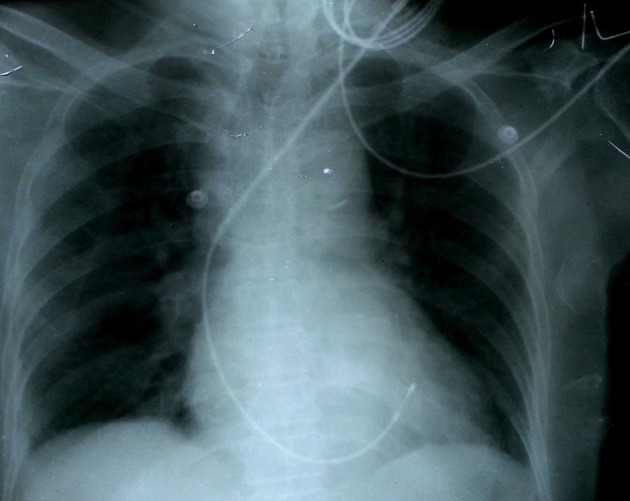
Chest X-ray in antero-posterior view showing the tip of the pacing lead directed towards left ventricle.
